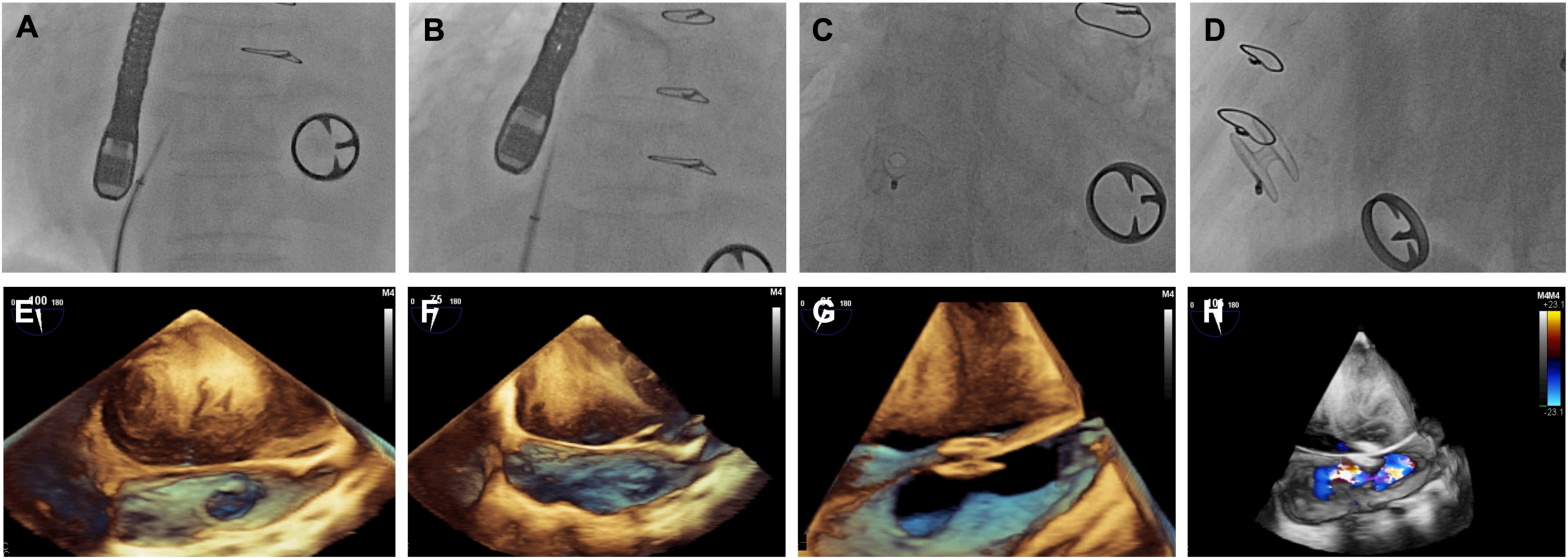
Images of D-shant device implantation. (A) x-ray and (E) transesophageal echocardiography (TEE) show the atrial septal position; (B) x-ray and (F) TEE guide atrial septal puncture; (C) x-ray and (G) TEE show release of the D-Shant device; and finally, (D) x-ray and (H) TEE color Doppler confirm the position of the D-Shant device and left-to-right shunting.
